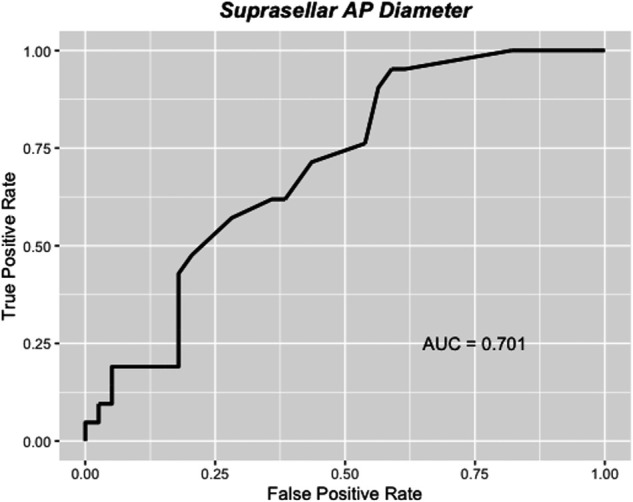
Receiver-operator characteristic curve demonstrating the performance of the suprasellar AP diameter as a predictor for reintervention after intraoperative MRI. The area under the curve was calculated to be 0.701, and the suprasellar AP diameter was found to significantly outperform a random classifier (*P* = .008). AUC, area under the curve; AP, anterior-posterior.
